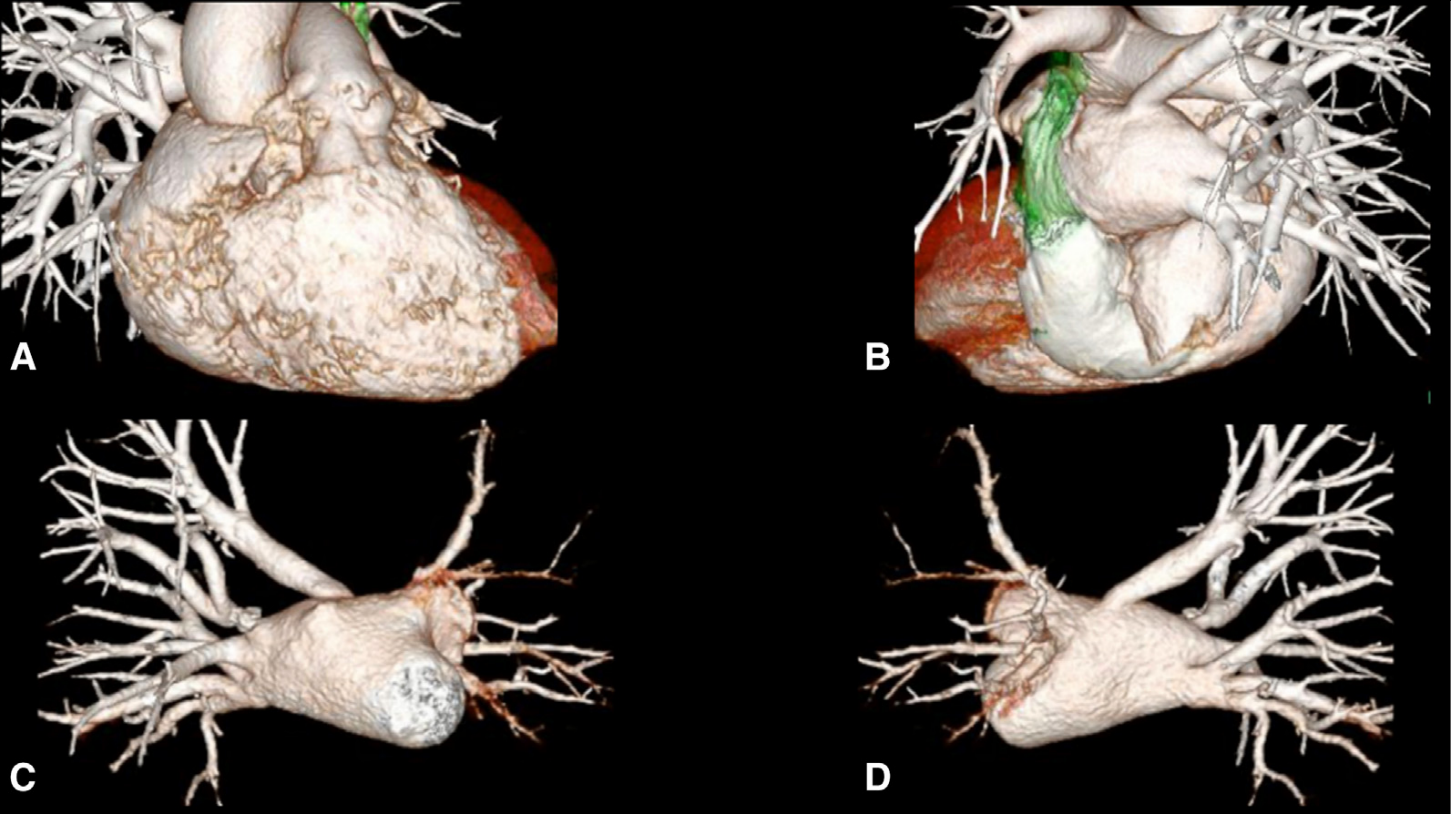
A and B, Preoperative computed tomography revealed complete occlusion of the PVs. The *green* structure represents the PLSVC. C and D, Postoperative computed tomography: The PV branches were patent.
